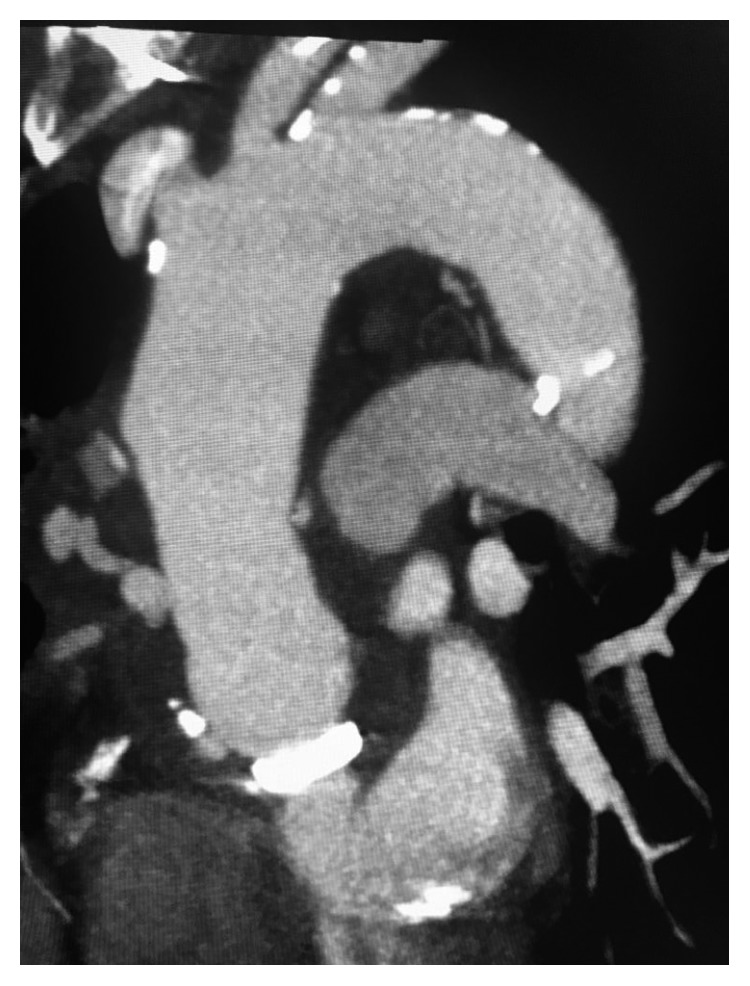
CTA showing pseudoaneurysmal degeneration of the aortic graft with fistulous flow to the right upper lobe.
